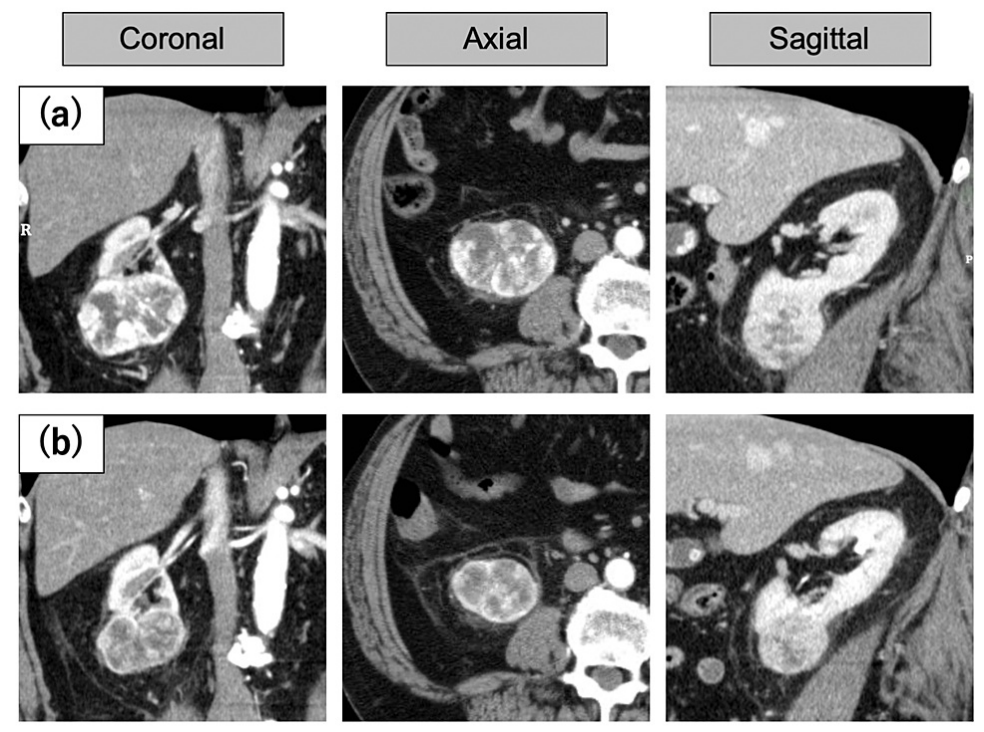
Case 3 renal tumor The contrast-enhanced CT of the renal tumor in Case 3 (a) shows the CT scan at the time of the initial diagnosis, revealing the tumor in direct contact with the iliopsoas muscle; (b) a CT scan before cryoablation after administration of molecular-targeted drugs. The tumor has undergone a reduction in both size and distance from the surrounding organs.
